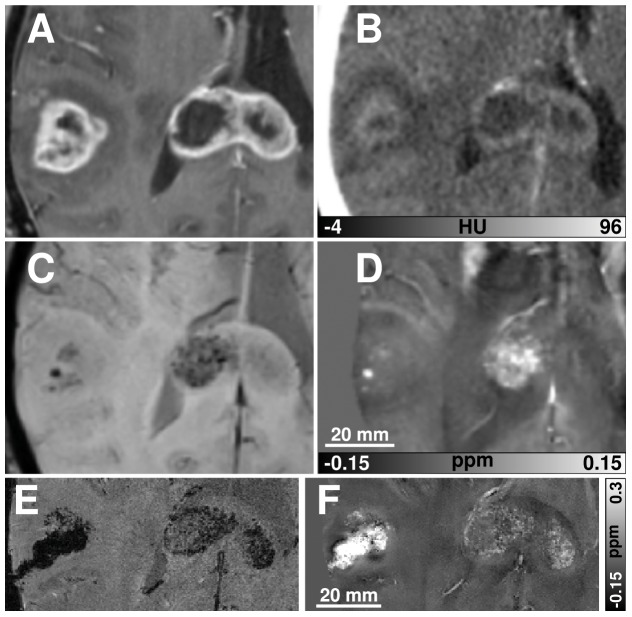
Imaging results of a 67-year-old female patient with a multilocular glioblastoma before radiochemotherapy. The contrast-enhanced T1-w image reveals the typical ring-enhancement (a), whereas the susceptibility weighted image shows intratumoral SBS in the temporal lesion and in the right part of the corpus callosum (c). Corresponding SBS on the susceptibility map (d) are hyperintense, indicating blood products as the biophysical source of the intratumoral SBS. The contrast-enhanced CT image (b), displaying a comparable section, proves these findings because no calcifications can be identified as possible sources of the tumor-related SBS. A single slice of the high-resolution 7 T GRE magnitude data and the corresponding susceptibility map are displayed in (e) and (f), respectively. Since the 7 T data were acquired 10 days after the routine MRI, biopsy of the right temporal lesion had been performed in the meantime.
